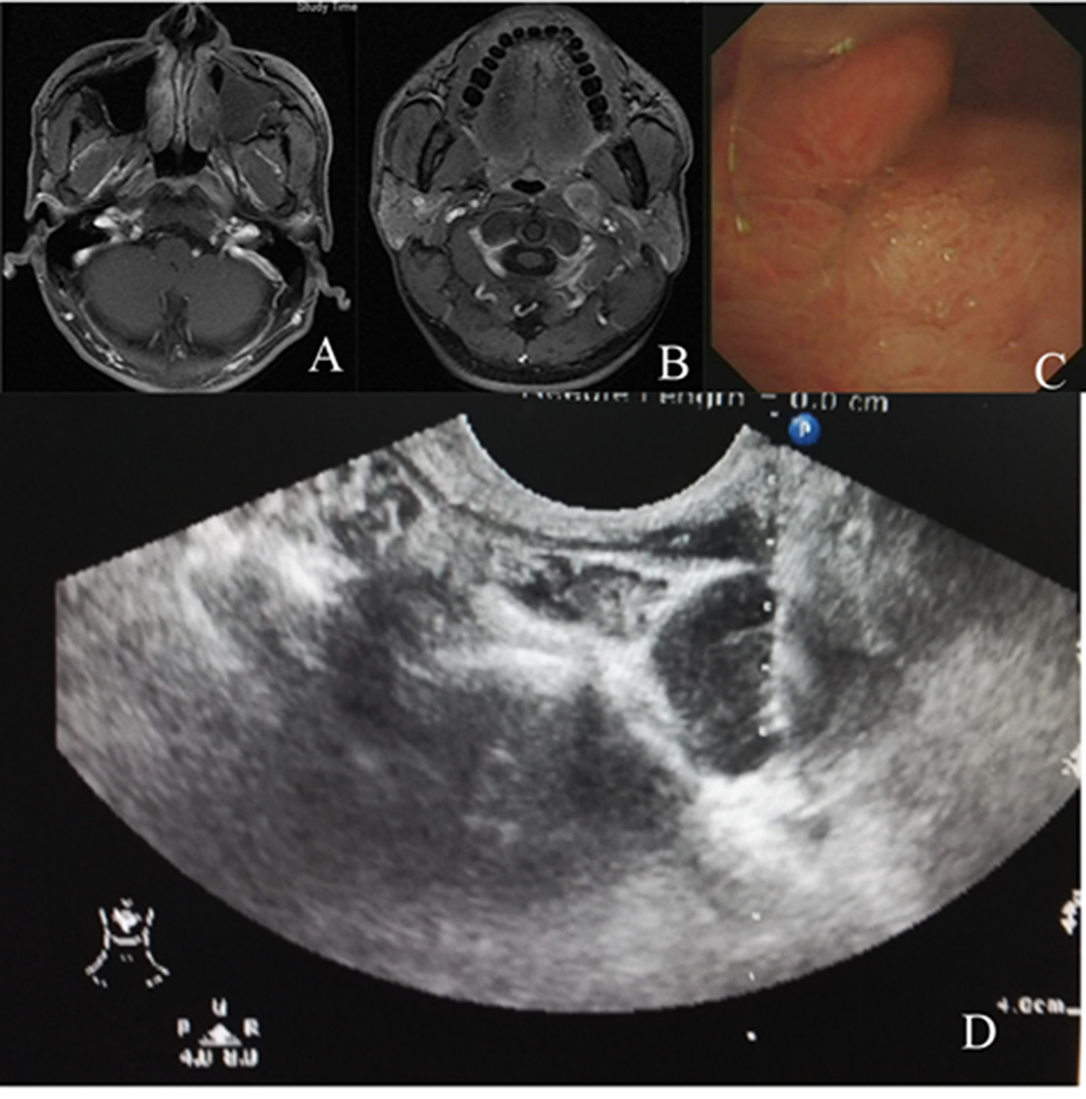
A male patient, 48y MRI (A) and nasopharyngo-fiberscope (C) revealed mucosa slightly thickening, but no evidence of malignancy was found after three times biopsy. Mass in the retropharyngeal space (B) was pathologically proven to be metastatic undifferentiated squamous carcinoma by transoral sonography-guided fine-needle aspiration (D).
